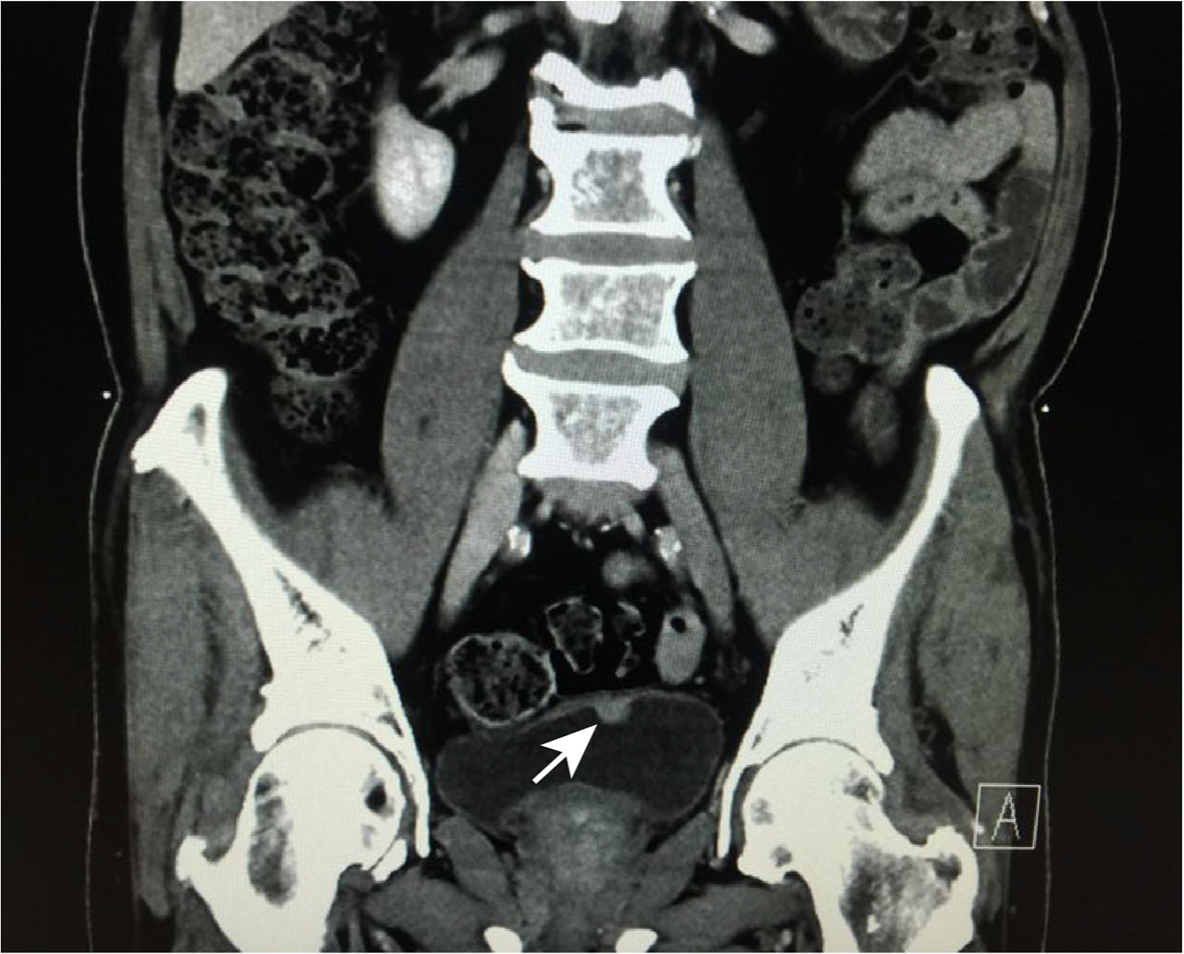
Pelvic computed tomography examination shows a nodular soft tissue shadow (arrow) at the left anterior wall of the bladder
